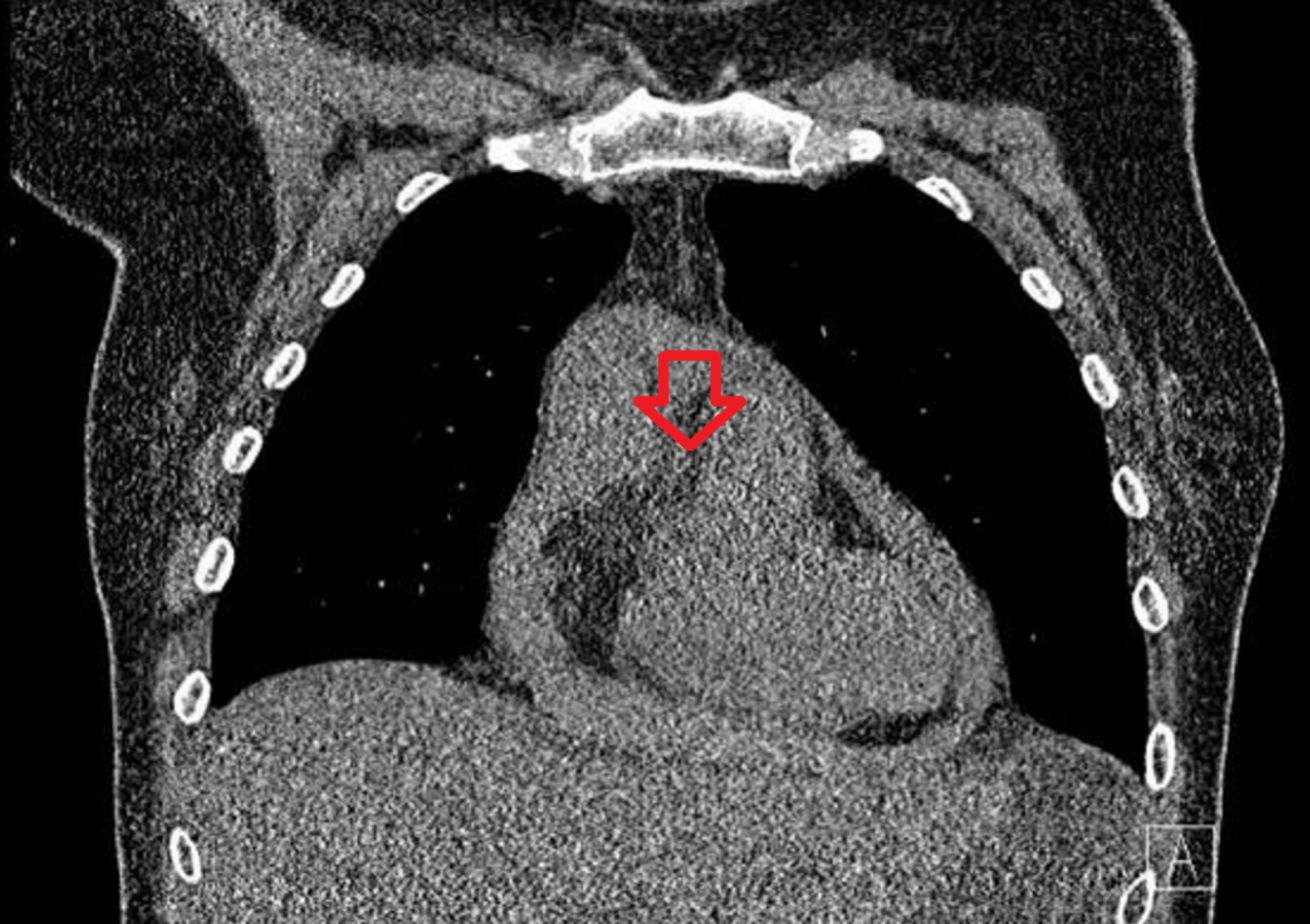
CT chest without contrast showed a large hemorrhagic appearing pericardial effusion and aneurysmal ectasia of the ascending aorta
